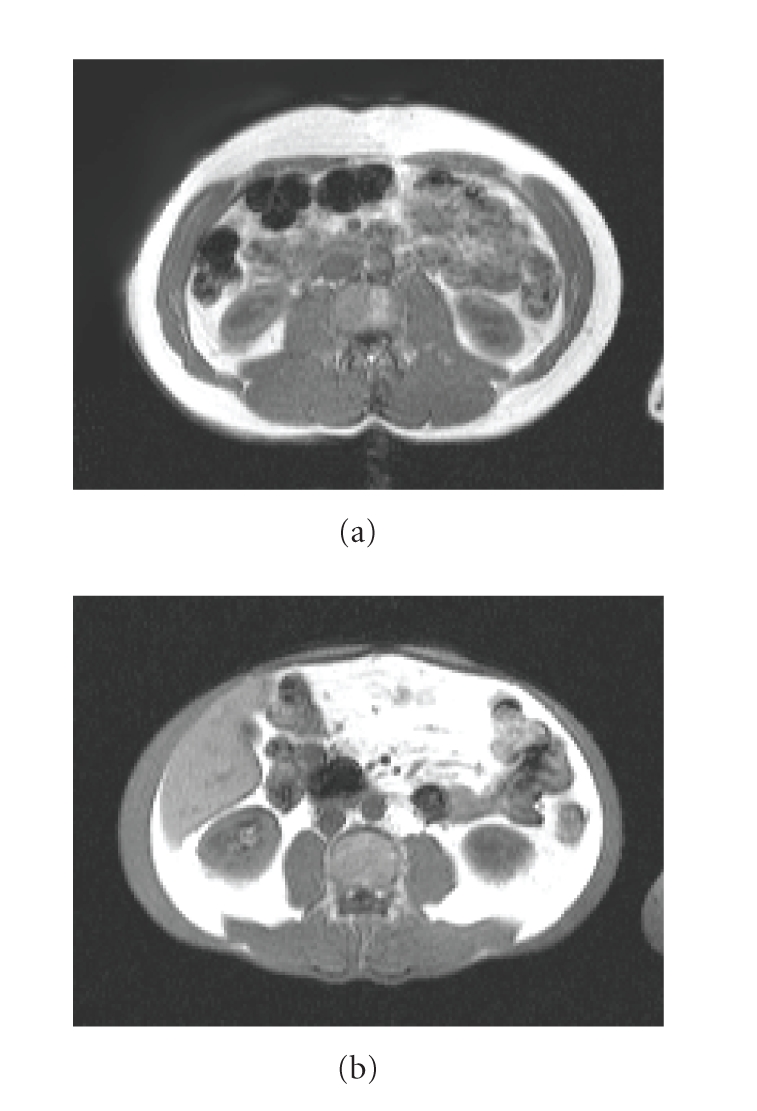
Abdominal magnetic resonance image (MRI) of a HAART-treated patient with normal fat distribution (a) and a patient with severe HAART-associated lipodystrophy with complete loss of subcutaneous fat and accumulation of intra-abdominal fat (b). Fat is shown white in these MRI images.
